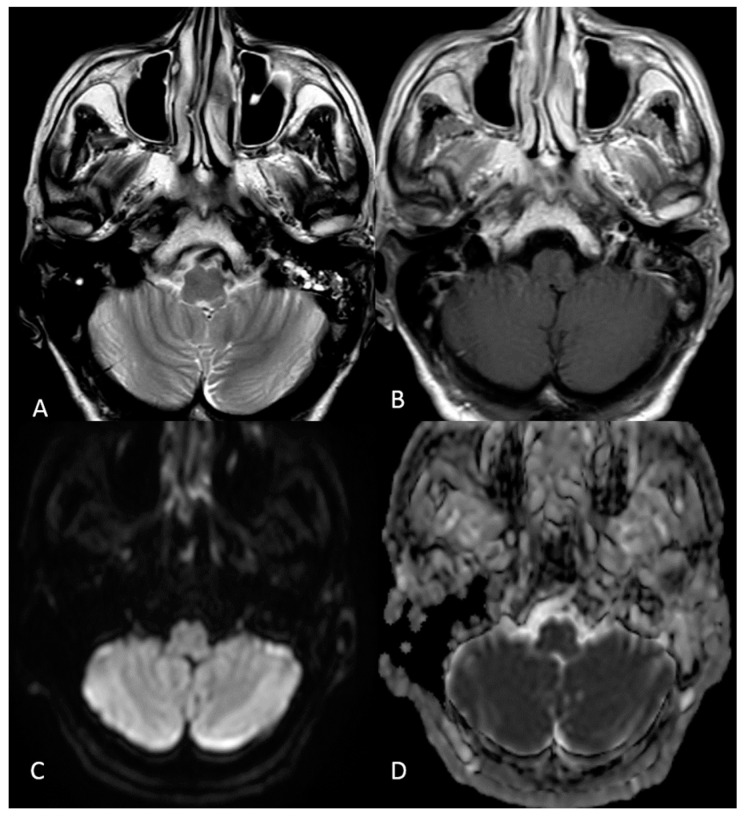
(A) T2-weighted image; (B) T1-weighted image post-contrast administration; (C,D) diffusion-weighted imaging b-1000 and apparent coefficient diffusion map, respectively. The lesion lacked contrast enhancement (B) and there was no diffusion restriction of water within the alteration (C,D).
